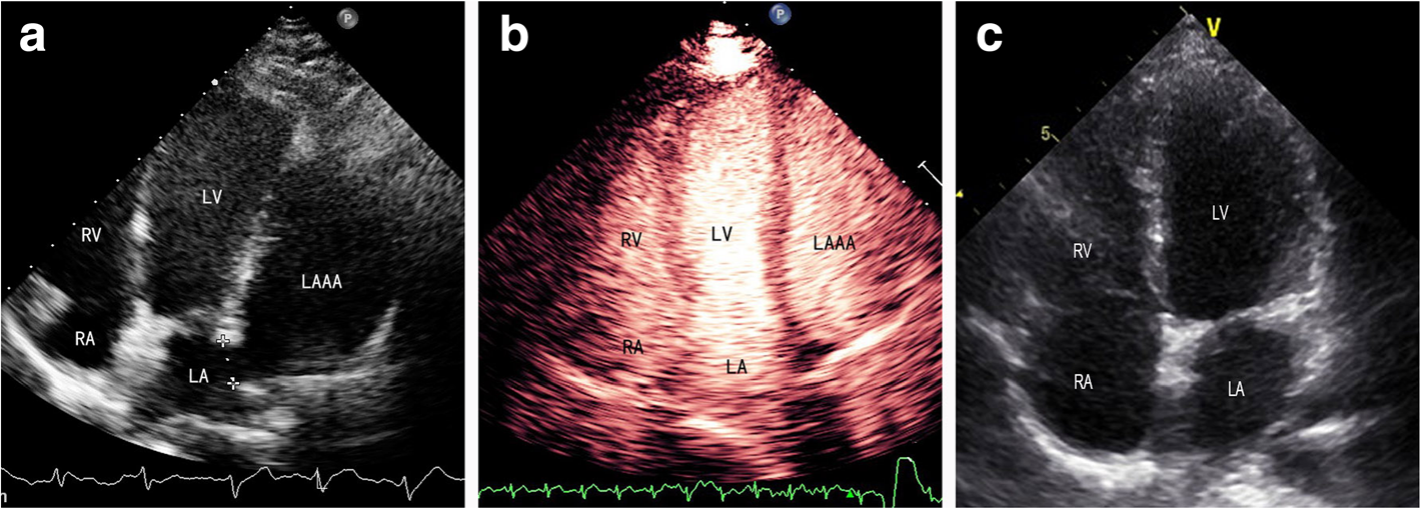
Transthoracic echocardiography showing the appendage aneurysm. a Conventional echocardiography showing a giant LAAA compressing the LV. b No thrombus was detected in the aneurysm by contrast echocardiography. c Echocardiography showing a normal-sized LA and that the aneurysm was no longer present
